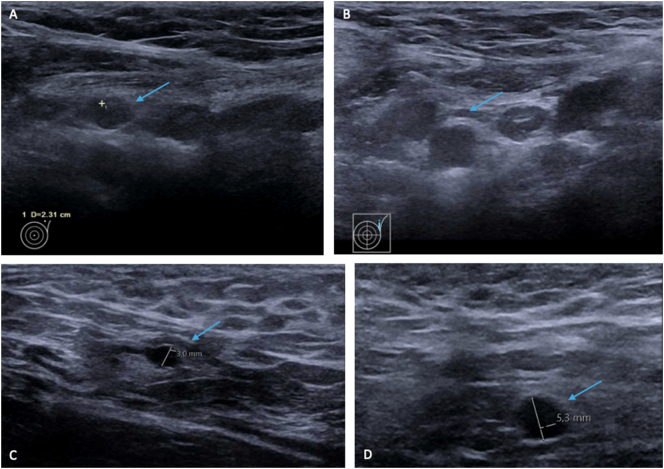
(A) Ultrasound showed four prominent left axillary lymph nodes (arrow), twenty-seven days after COVID-19 vaccination. (B) Ultrasound impressed consistent with previous ultrasound (arrow), ninety-six days after COVID-19 vaccination. (C) Ultrasound showed a right axillary solitary lymph node (arrow), nine days after COVID-19 vaccination. (D) Ultrasound showed multiple prominent left axillary lymph nodes (arrow), four days after COVID-19 vaccination.
